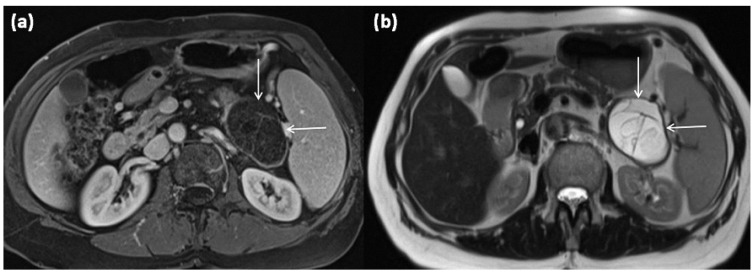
MRI of a 67-year-old female patient with T1w sequence after gadolinium-based contrast administration (a) and T2w sequence (b). A large multicystic mass of the pancreatic tail (arrows) with tiny septa in T2w, as well as mild contrast enhancement of each septa, is seen.
